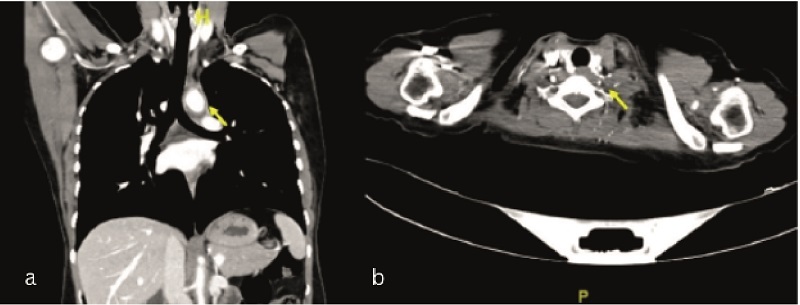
CT aortogram showing thickening and vascular occlusion of the thoracic aorta (a) and left vertebral artery (b) in coronal and axial scans, respectively, as marked by the arrows.
